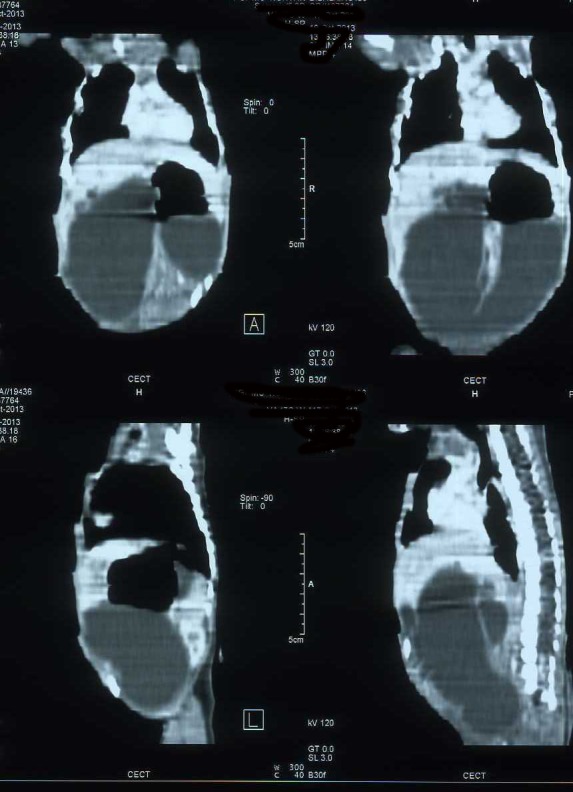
Figure 2: CT scan of the abdomen showing a dilated fluid filled gut loop.
